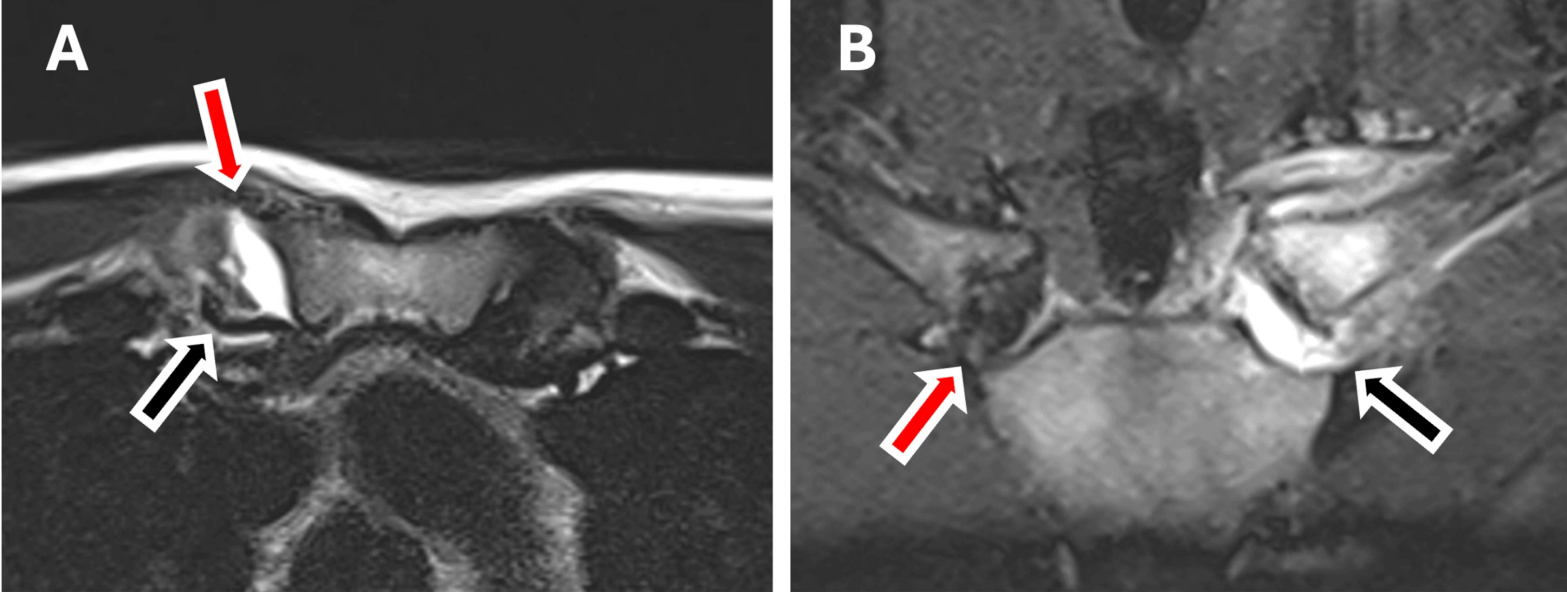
Magnetic resonance imaging scan A. Axial T2 magnetic resonance imaging arthrogram of both sternoclavicular joints. On the left, the gadolinium can be seen in the joint, penetrating through the anterior capsule (red arrow). The intra-articular disc lies posterior to the medial end of the clavicle, still attached to the sternum (black arrow); B. Coronal T2 magnetic resonance imaging arthrogram of both sternoclavicular joints. On the left, the joint space is filled with the gadolinium dye, and the disc is absent (black arrow). On the right, the intra-articular disc can be seen between the sternal and clavicular joint surfaces (red arrow).
